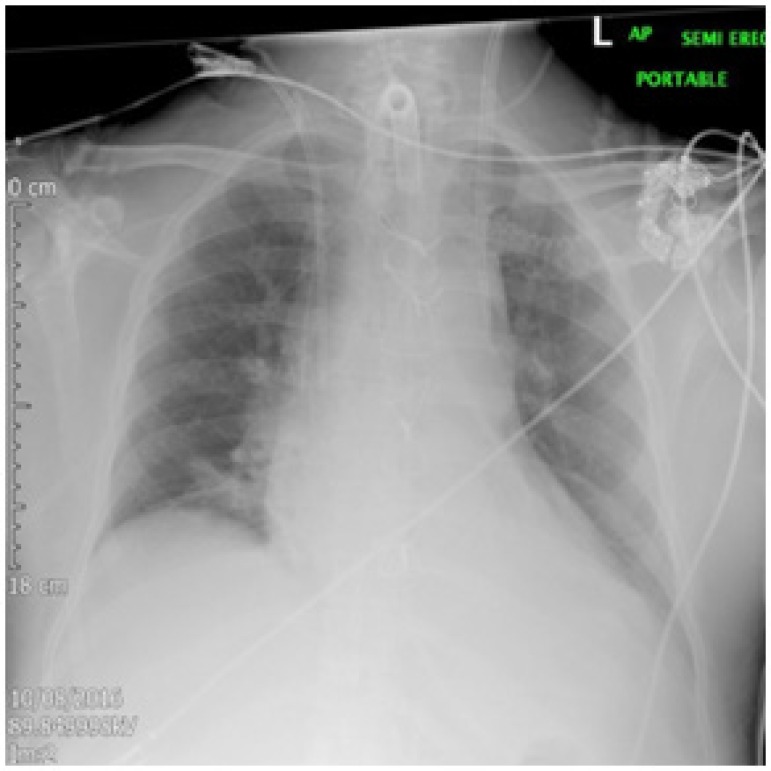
The chest x-ray shows mal-positioned dialysis catheter.
